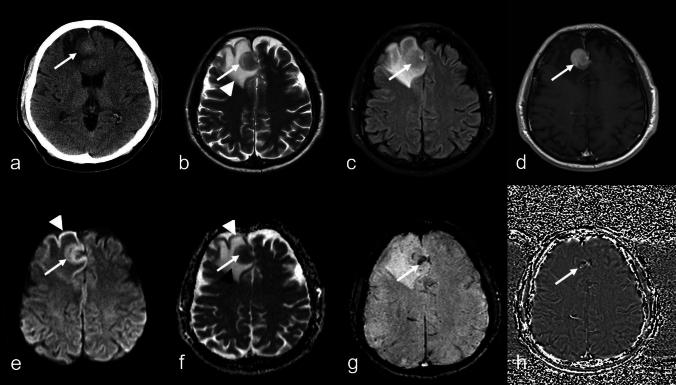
62-year-old man with primary large B-cell lymphoma of immune-privileged sites (IP-LBCL), negative for Epstein–Barr virus. a: Axial CT showing a slightly hyperdense intraaxial lesion (arrow); b–h: MRI showing an inhomogeneous hypointense cortical and subcortical solid lesion (b, c: T2-weighted imaging (WI), fluid-attenuated inversion recovery, arrows) with homogeneous contrast enhancement (d: T1WI; arrow) and restricted diffusion (e: diffusion-WI, b = 1000 s/mm^2^; f: apparent diffusion coefficient (ADC) map, mean ± SD: 0.69 ± 0.14 × 10⁻^3^ mm^2^/s, arrow; surrounding vasogenic edema (f; black arrowhead; ADC value mean ± SD: 2.2 ± 0.07 × 10⁻^3^ mm^2^/s; g: susceptibility-WI: confluent signal loss (arrow); h: phase image (arrow). Note the bandlike cortical diffusion restriction in the right frontal region (e, f; arrowheads), consistent with focal epileptic seizures
